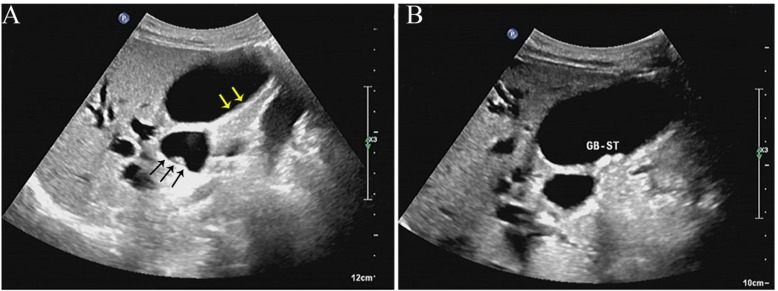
Ultrasonographic findings of gallbladder iNKLPD. (A) Transverse ultrasound image reveals focal, asymmetrical wall thickening of the gallbladder (yellow arrows). A corresponding hypoechoic, submucosal tumor-like lesion is identified at the gallbladder neck (black arrows). (B) A separate ultrasound image demonstrates multiple hyperechoic foci with posterior acoustic shadowing within the gallbladder lumen, consistent with cholelithiasis. iNKLPD, indolent natural killer-cell lymphoproliferative disorder.
